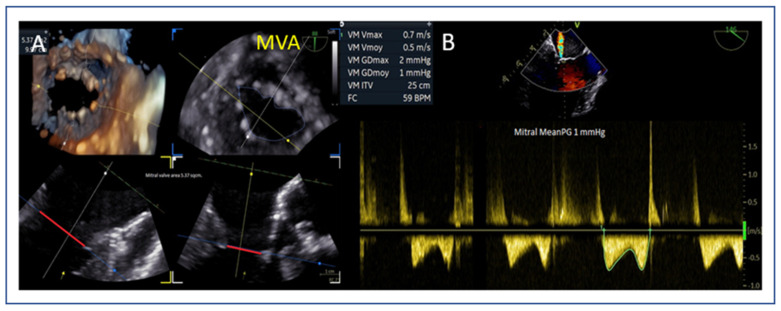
(A) Mitral valve area measurement using MPR. (B) Baseline mean mitral valve gradient using CW Doppler. MVA: mitral valve area.
